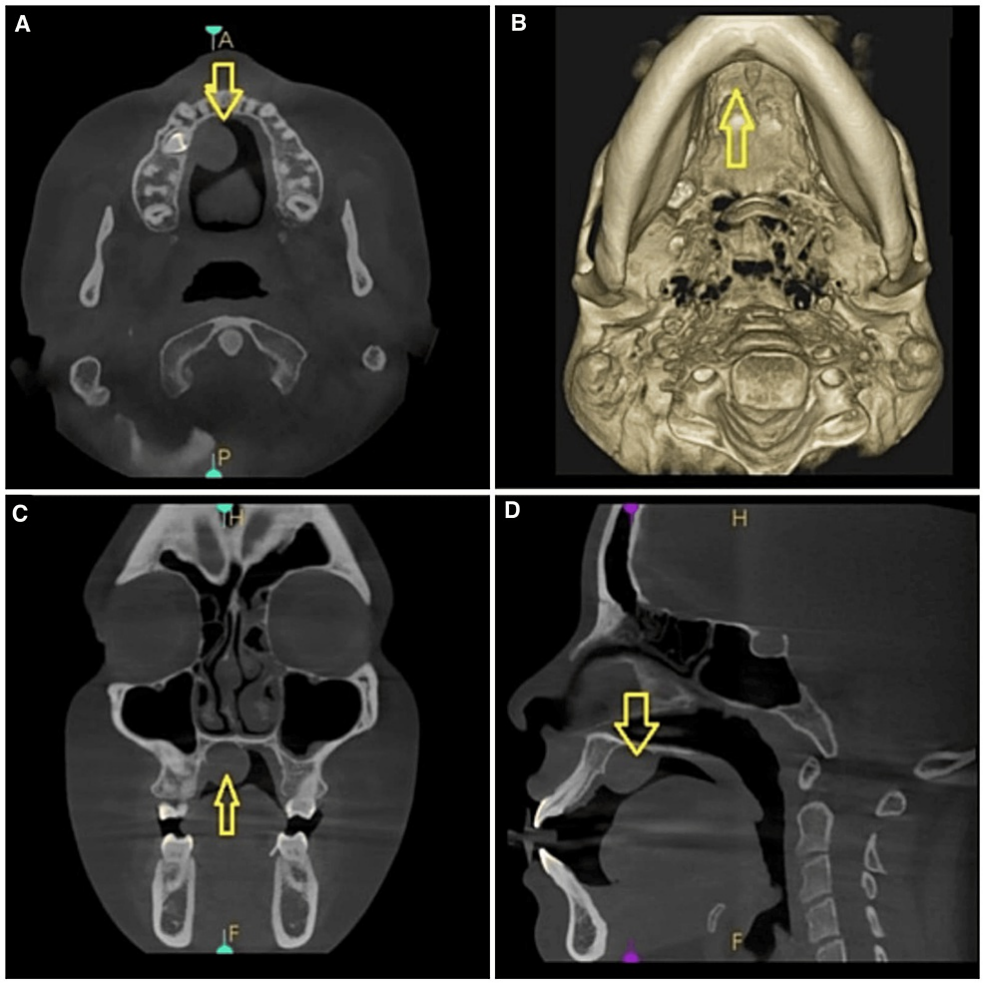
Cone-beam computed tomography sections depicting the lesion (arrow) A: axial section; B: three-dimensional contrast section; C: coronal section; D: sagittal section
